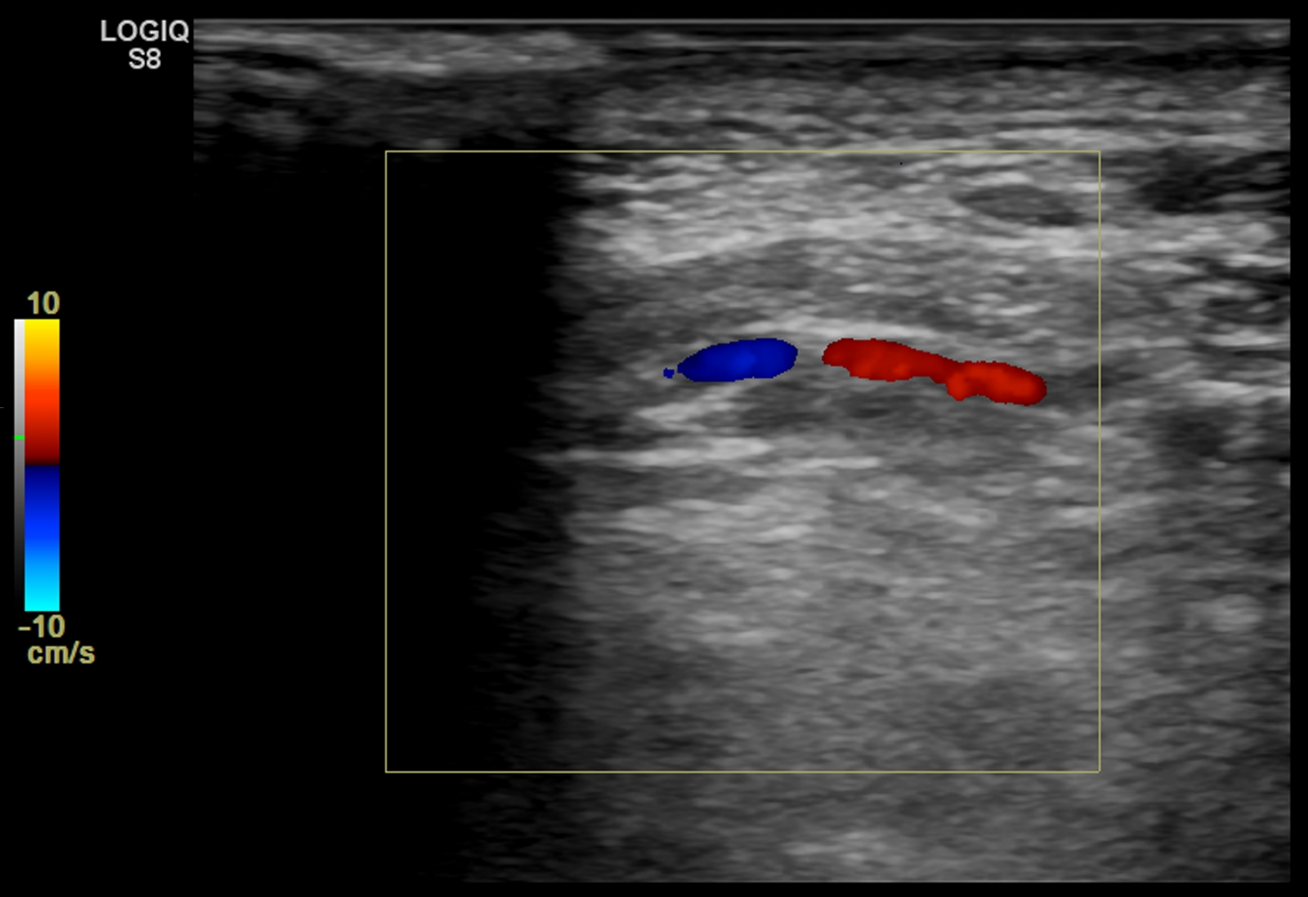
Duplex ultrasound image from the left lateral neck demonstrates the left vertebral artery near the C2 vertebra.
